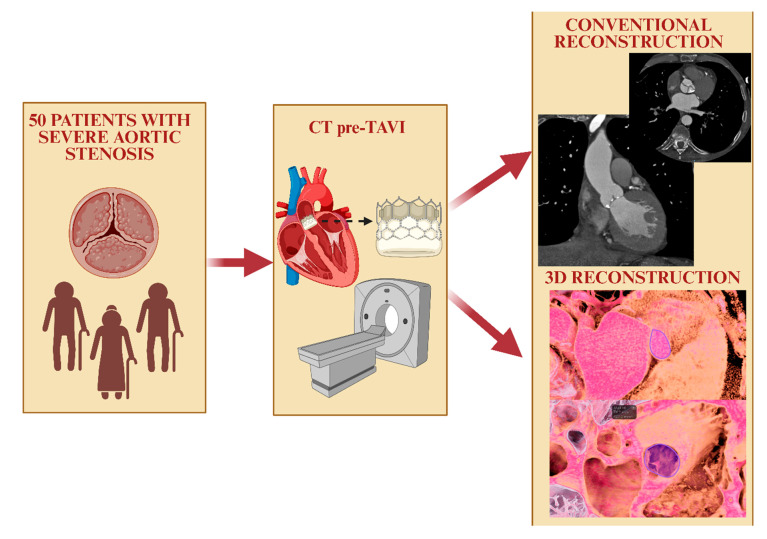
Comparison of conventional and 3D virtual reality reconstruction from pre-TAVI CT in severe aortic stenosis.
